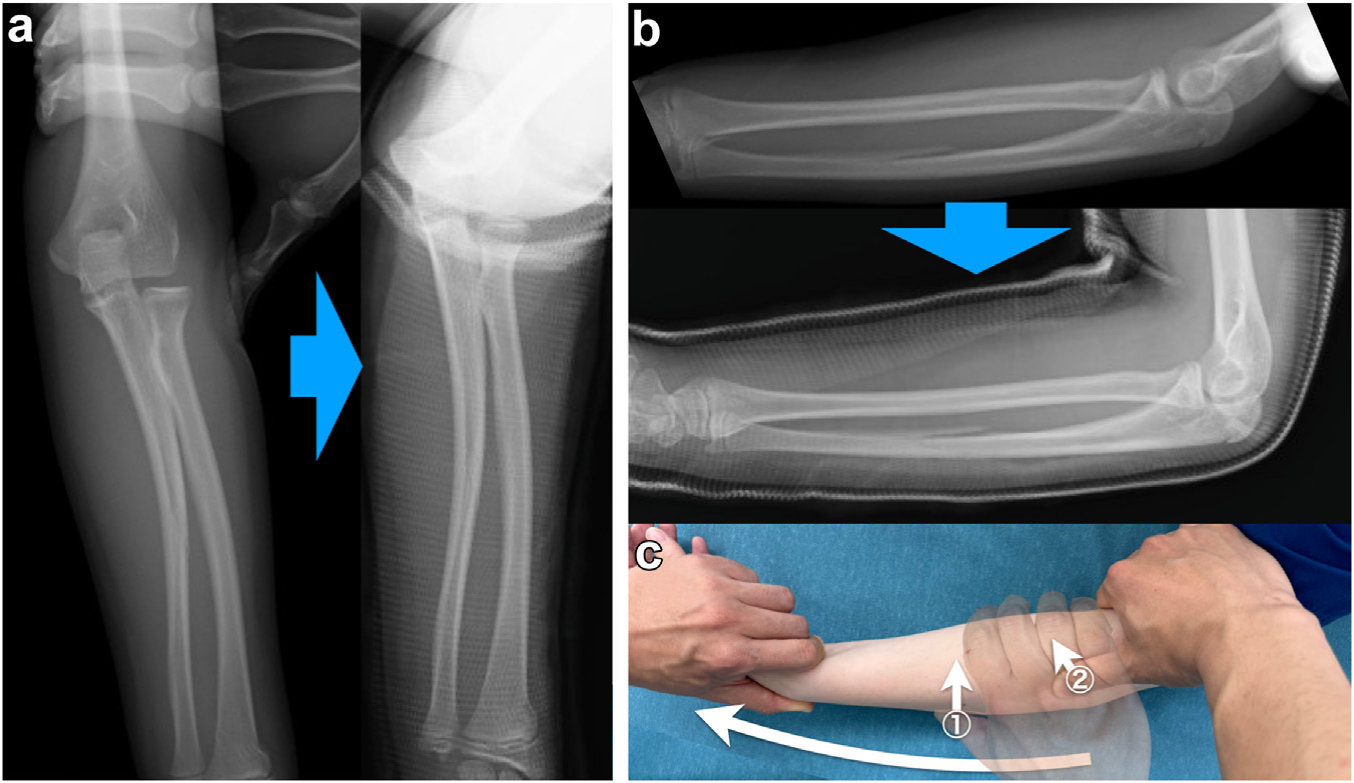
Fluoroscopic and radiographic images during closed reduction. (a) Antero–posterior view. (b) Lateral view. The radiograph after above-elbow cast was inaccurate and insufficient for evaluating the radial head dislocation. (c) Demonstration of the closed reduction. During forearm traction, the angulation of the ulna shaft was corrected by pushing the ulna from the posterior side (*white arrow 1*), followed by correcting the dislocation of the radial head by applying pressure from the lateral side (*white arrow 2*).
